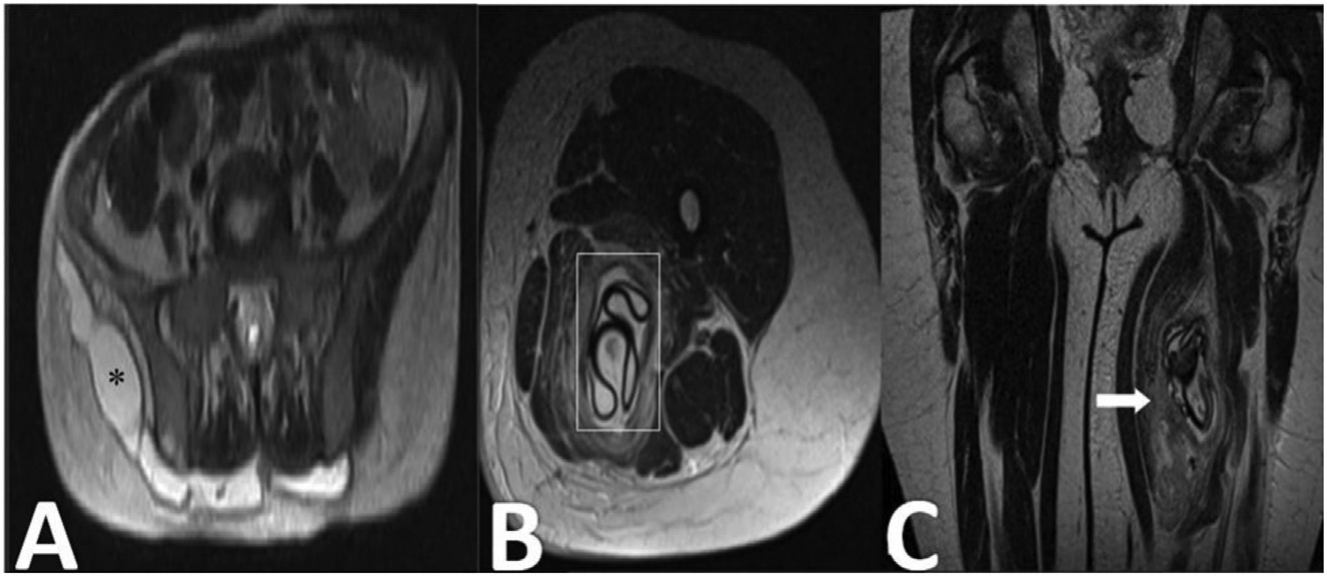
Axial T2 fat-sat MRI (A) of the pelvis shows an extensive cystic lesion in the gluteal soft tissue with low-signal wall (Asterisk). The lesion proved to be hydatid disease. Axial (B) and coronal (C) T2-weighted MRI of the thigh in a middle-aged man shows the typical layered appearance hydatid cyst (rectangle) in a thigh muscle. Note the presence of edema (arrow) adjacent to the hydatid cyst. (Photos are from our own patient(s) and they had not been published previously elsewhere.).
